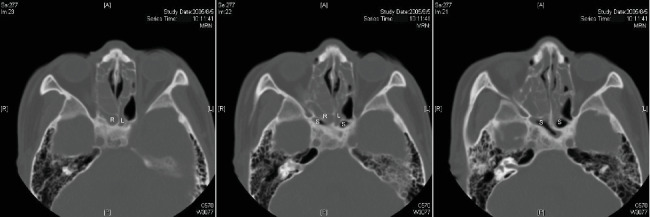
Initial presentation of the bilateral diseased sphenoethmoidal cells in 2005. Consecutive CT images of the patient who developed visual loss. R: right sphenoethmoidal cell; L: left sphenoethmoidal cell; S: sphenoid sinus.
